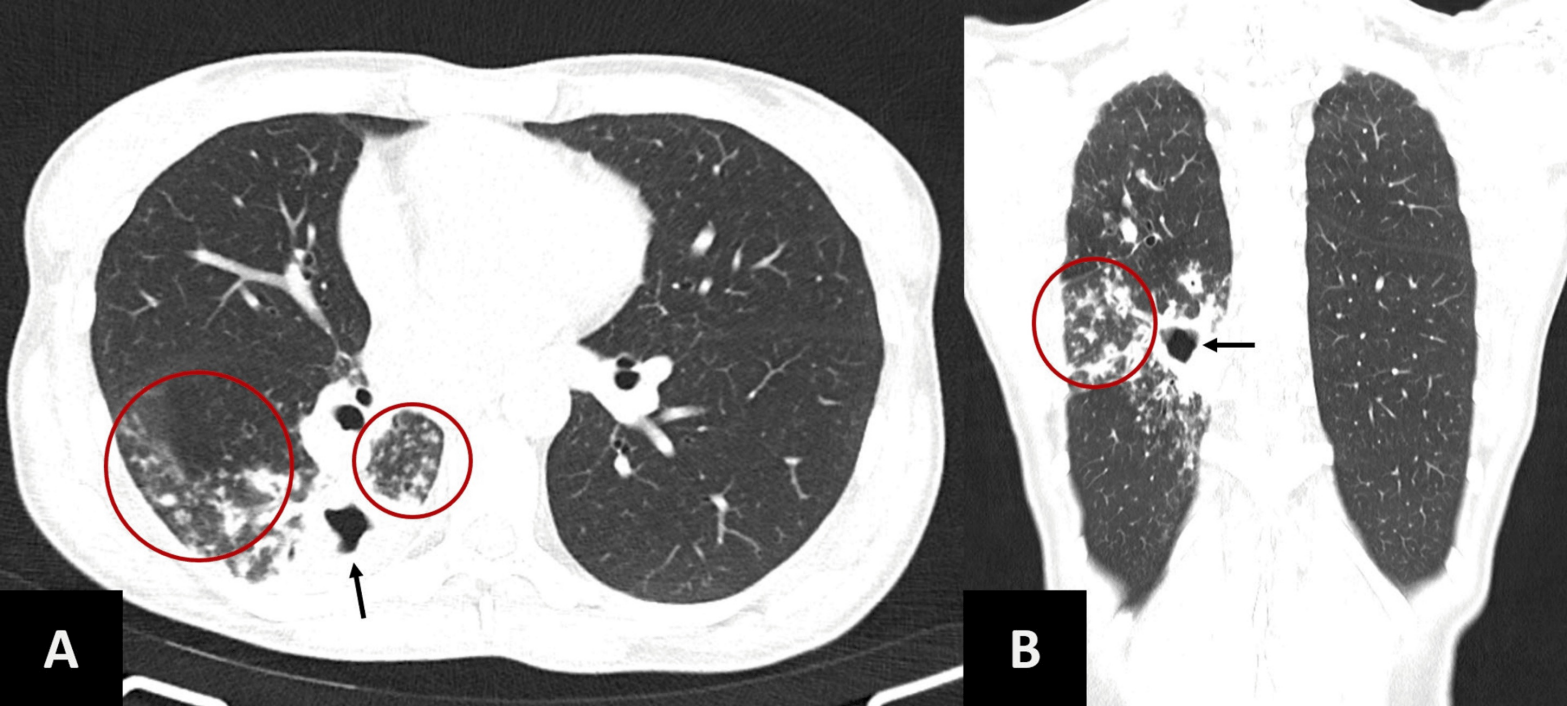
Chest CT scan A) Axial view and B) Coronal view. Scans evidencing a cavitated lesion in the upper segment of the right lower lobe (arrows) and ground glass infiltrates around the lesion (circles) CT - Computed tomography
